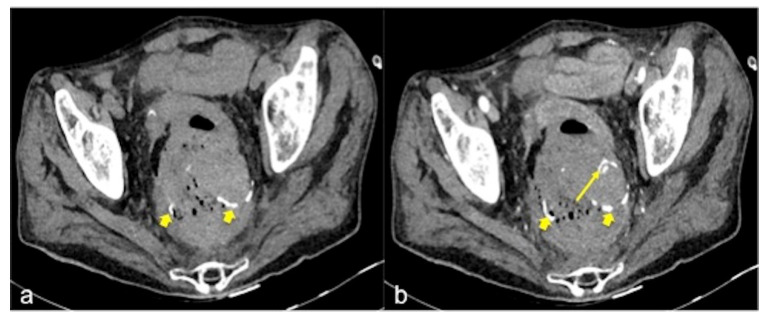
CT multiphasic study of haemorrhage at the surgical site of colorectal anastomosis. The pre-contrast CT axial image (a) shows spontaneous hyperdensity of metallic clips (small arrow). The arterial phase (b) shows another hyperdense spot (long arrow) near the anastomosis and is suggestive of active bleeding.
